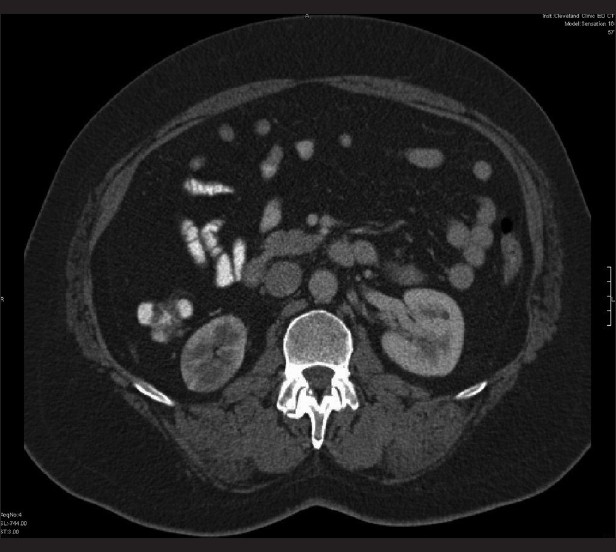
Coronal recon computed tomography enhanced with IV contrast exhibiting an irregular hypodense area of superior pole of the right kidney consistent with hypoperfusion
